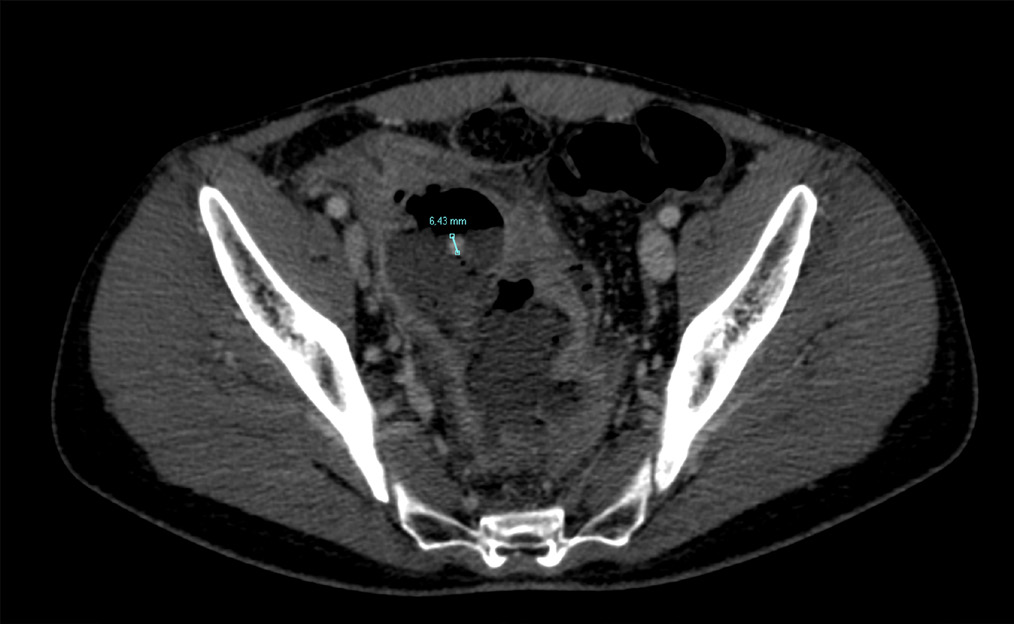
Axial post-contrast CT scan in portal venous phase shows a subcentimetric appendicolith inside the abscess.
